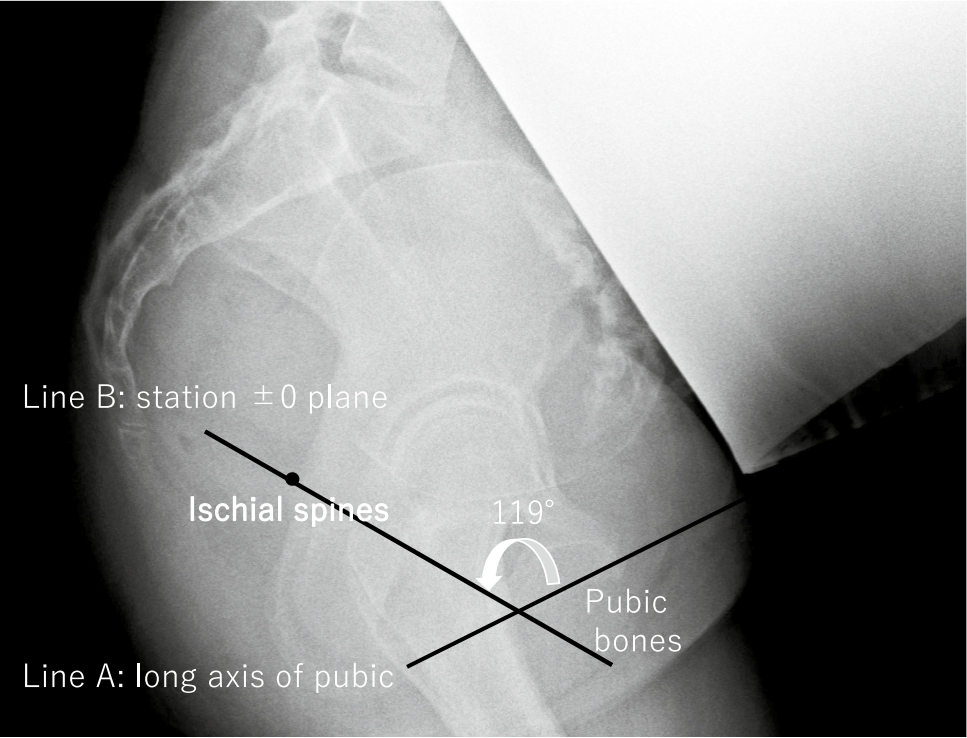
Identification of station ± 0 in pelvic radiography using the Guzman method. On Guzman X-ray picture, the line from the ischial spine to the lower margin of the pubic bone (line B) represents station ± 0. Line A is the long axis of the pubic symphysis. Line B passing through the ischial spine and the lower margin of the pubic bone is defined as “station ± 0”. The angle between lines A and B was measured. We detected the 119° line from line A as corresponding to station ± 0 as determined using digital vaginal examination (DVE) in Japanese women
